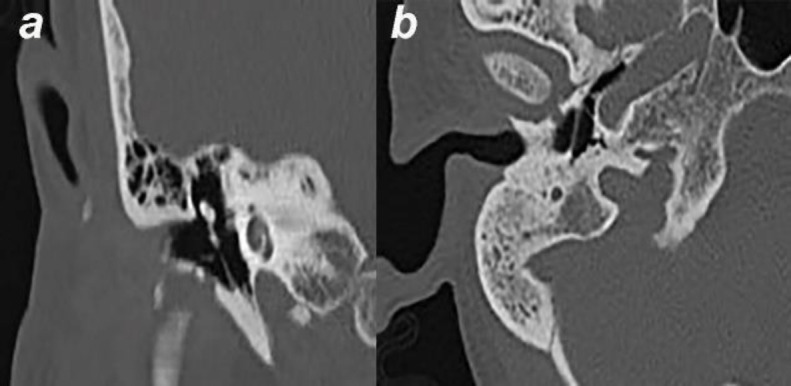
Computed tomography images showing the foramen of Huschke defect in the tympanic bone on the right side. (a) Coronal oblique reformatted image and (b) axial bone window
